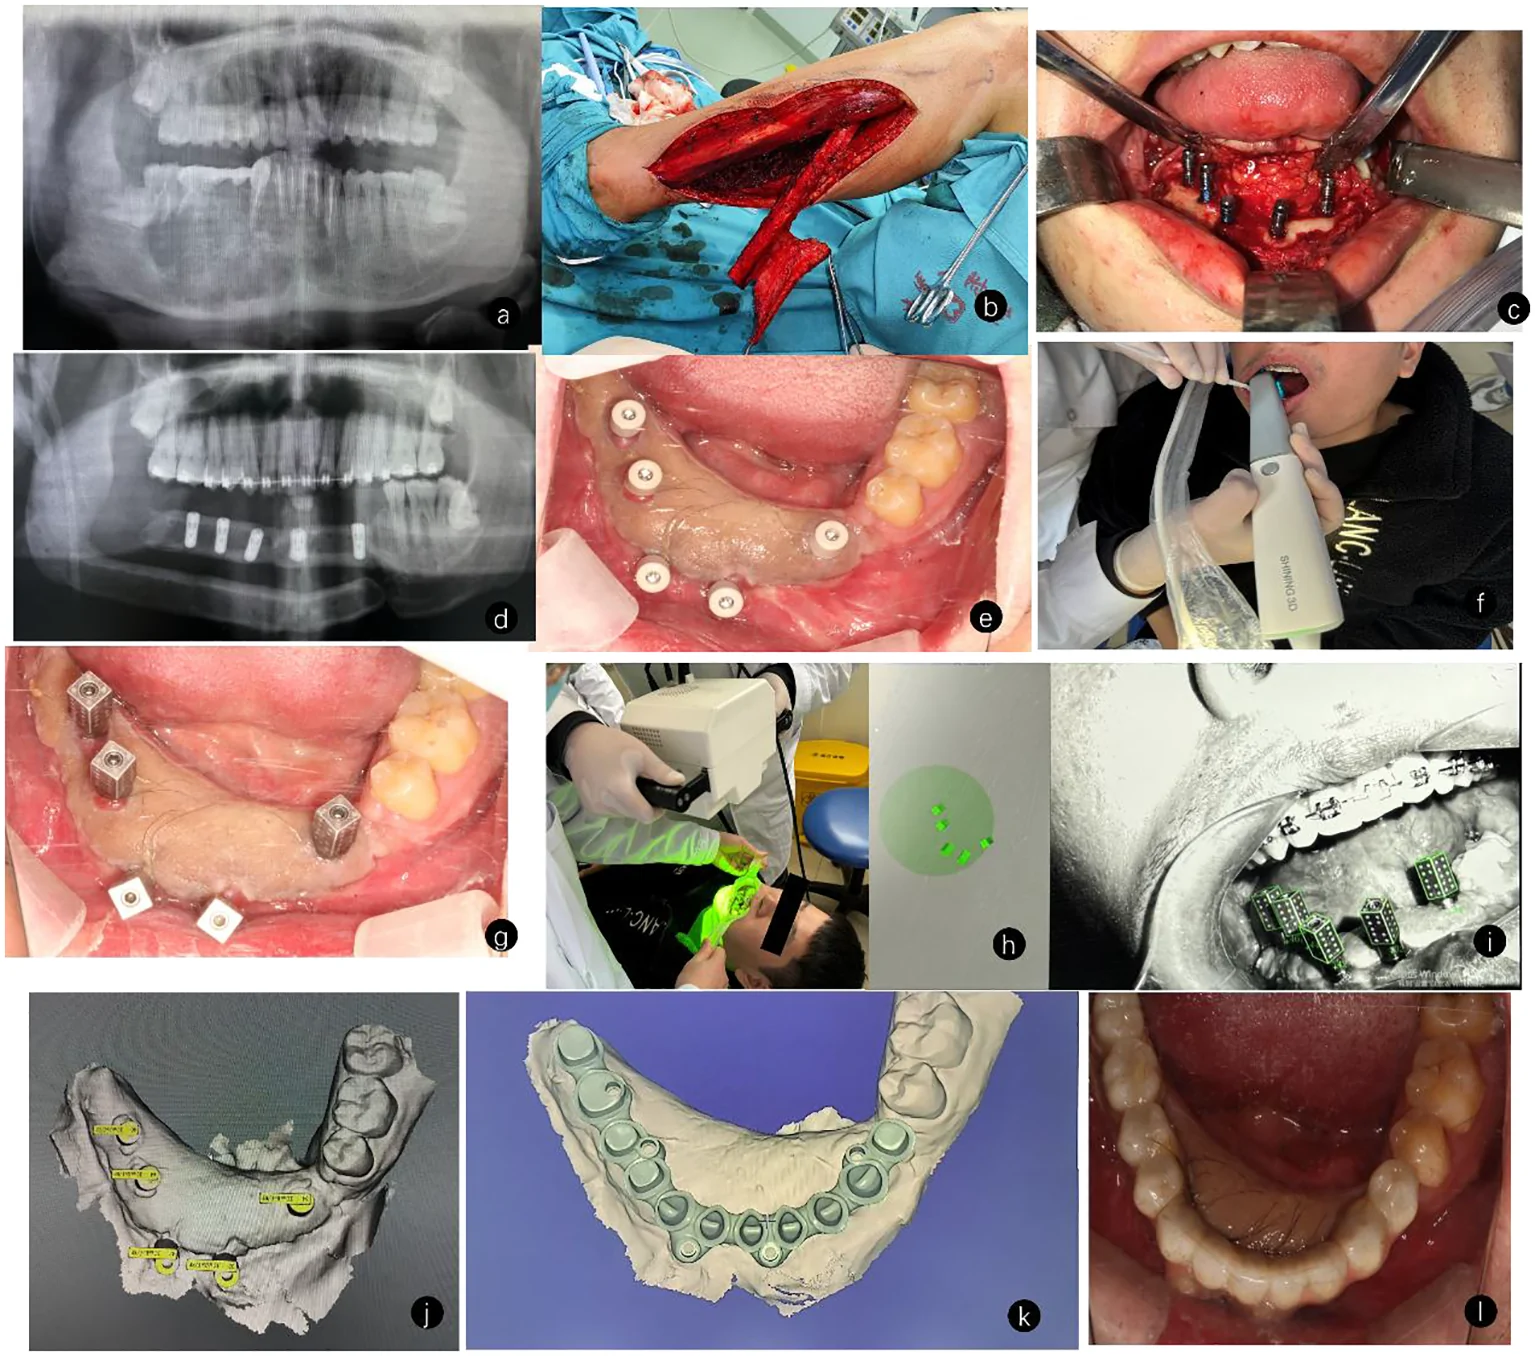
Representative case (A) Panoramic radiograph (B) Intraoperative harvesting of a fibula flap for mandibular reconstruction. (C, D) Implant placement and the corresponding panoramic radiograph. (E–I) Acquisition of digital data using SPG combined with IOS. (J) Matching iCamPosition spatial data with soft tissue intraoral scan data. (K) Digital design of implant prosthesis. (L) Intraoral view after implant denture placement.
